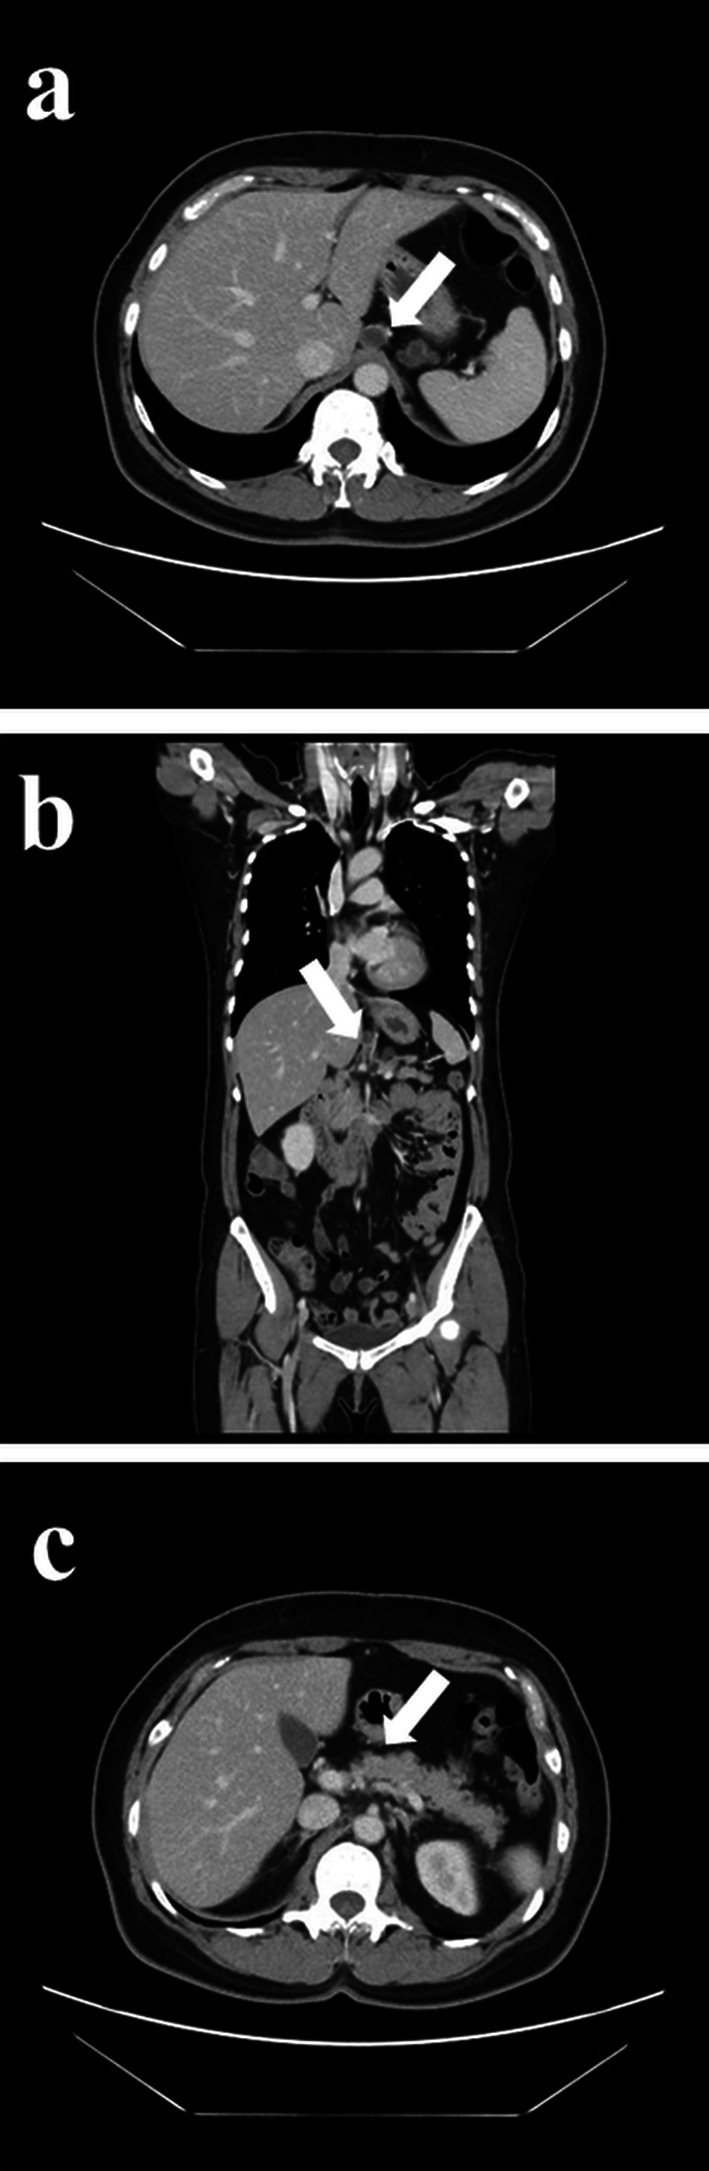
CT images after neoadjuvant chemotherapy. a The shrinkage of both GDC and cystic formation of the pancreatic duct was observed (white arrow). b The fistula between the GDC and the pancreas could be observed (white arrow). c The tumor became unclear after neoadjuvant chemotherapy (white arrow)
